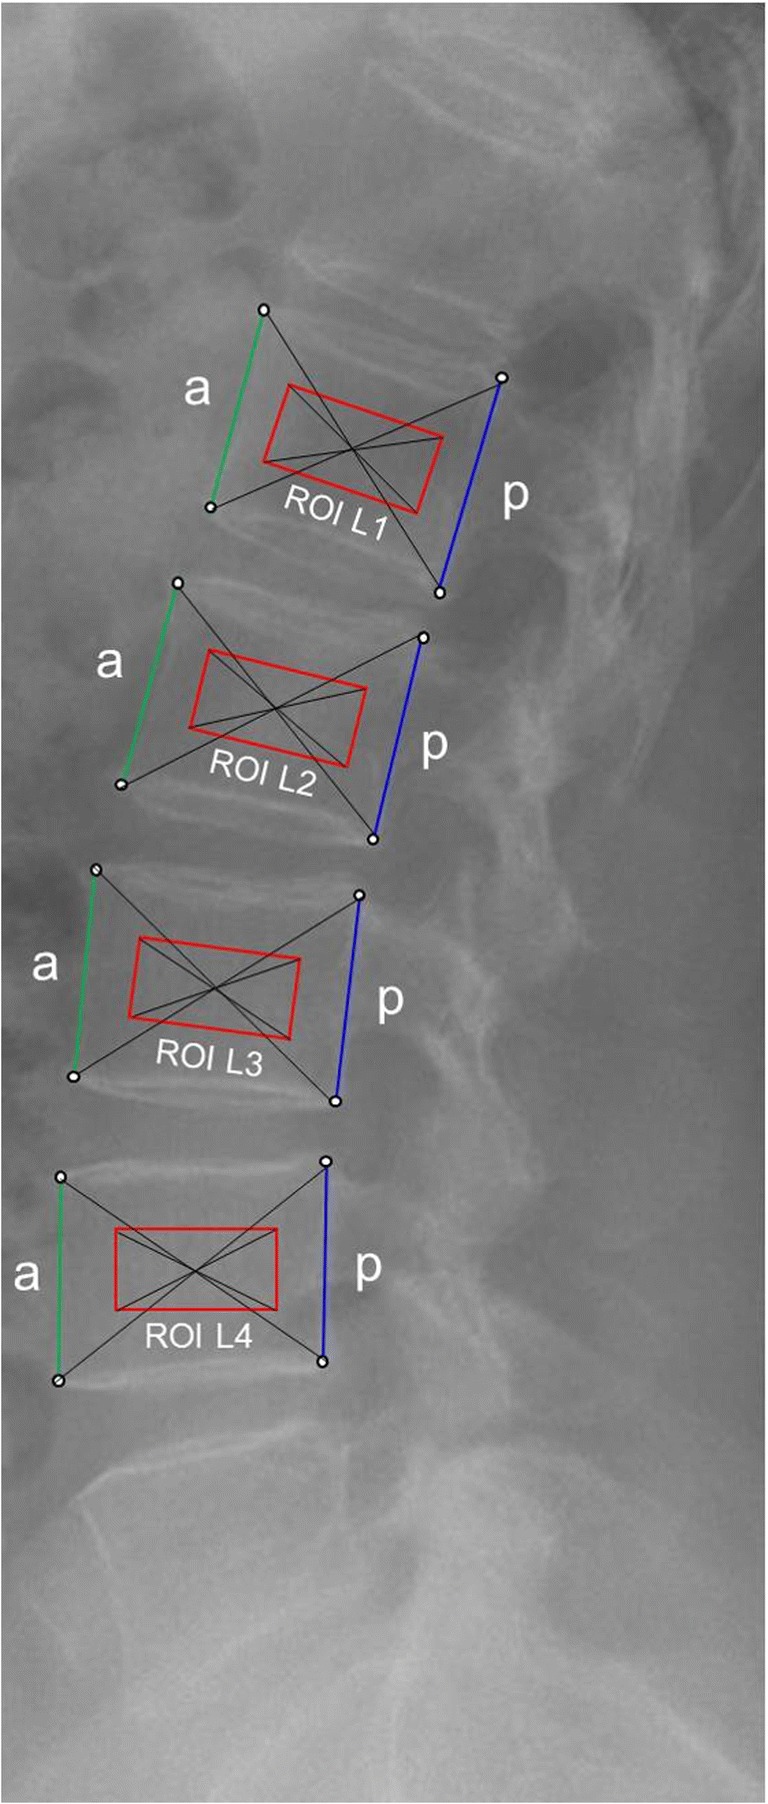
Semi-automatic ROI placement for measurement of BSV. Anterior (a) and posterior (b) edges of each vertebra were marked manually. ROIs sized 28 × 14 mm (*red rectangle*) were then set automatically so that the center of each vertebra was congruent with the center of the according ROI. The radiograph belongs to patient #3 (Table 1) and was taken at the screening visit in 2004
